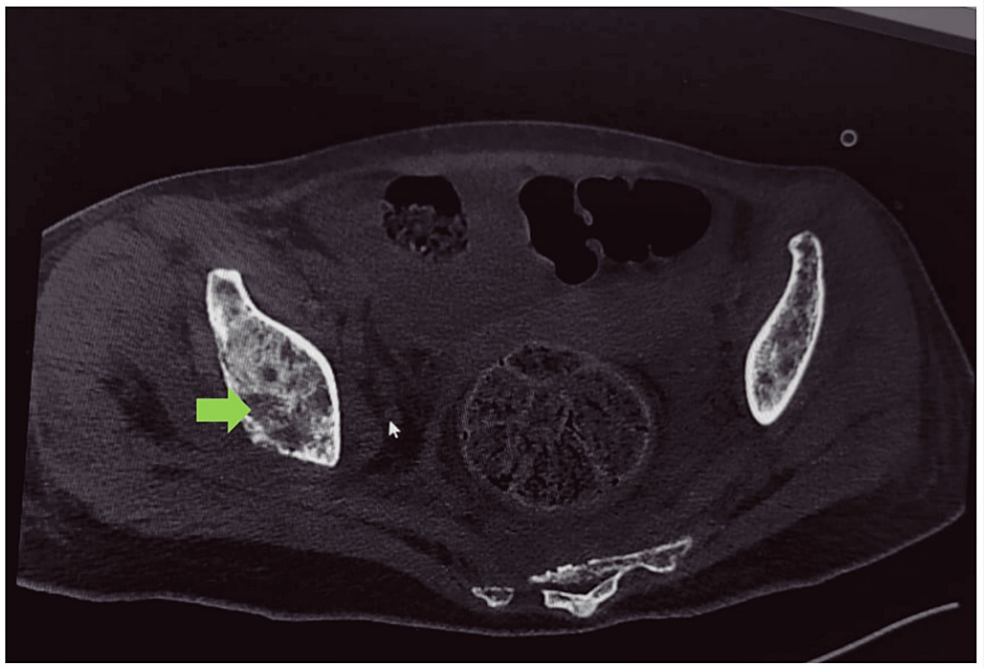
Non-contrast computed tomography showing multiple lytic lesions with aggressive periosteal reaction and a large soft tissue component epicenter in the right ischium (green arrow).
